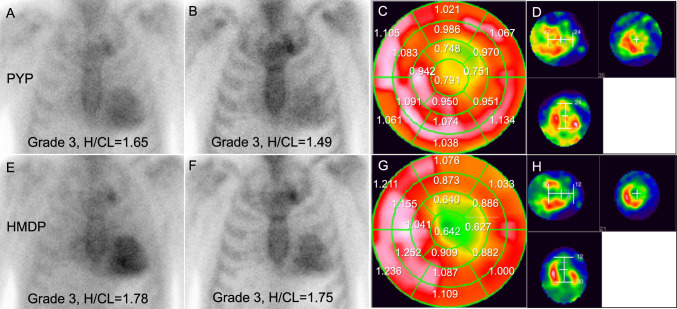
Representative case of a man in his 70 s with transthyretin cardiac amyloidosis. Upper row (A–D): PYP; lower row (E–H): HMDP. From left to right: planar images at 1 h, planar images at 3 h, polar maps, and representative SPECT slices. Both tracers demonstrated intense myocardial uptake. Polar maps (C, G) display relative segmental uptake (RSU) values. *PYP: pyrophosphate; HMDP: hydroxymethylene diphosphonate; SPECT: single photon emission computed tomography*
